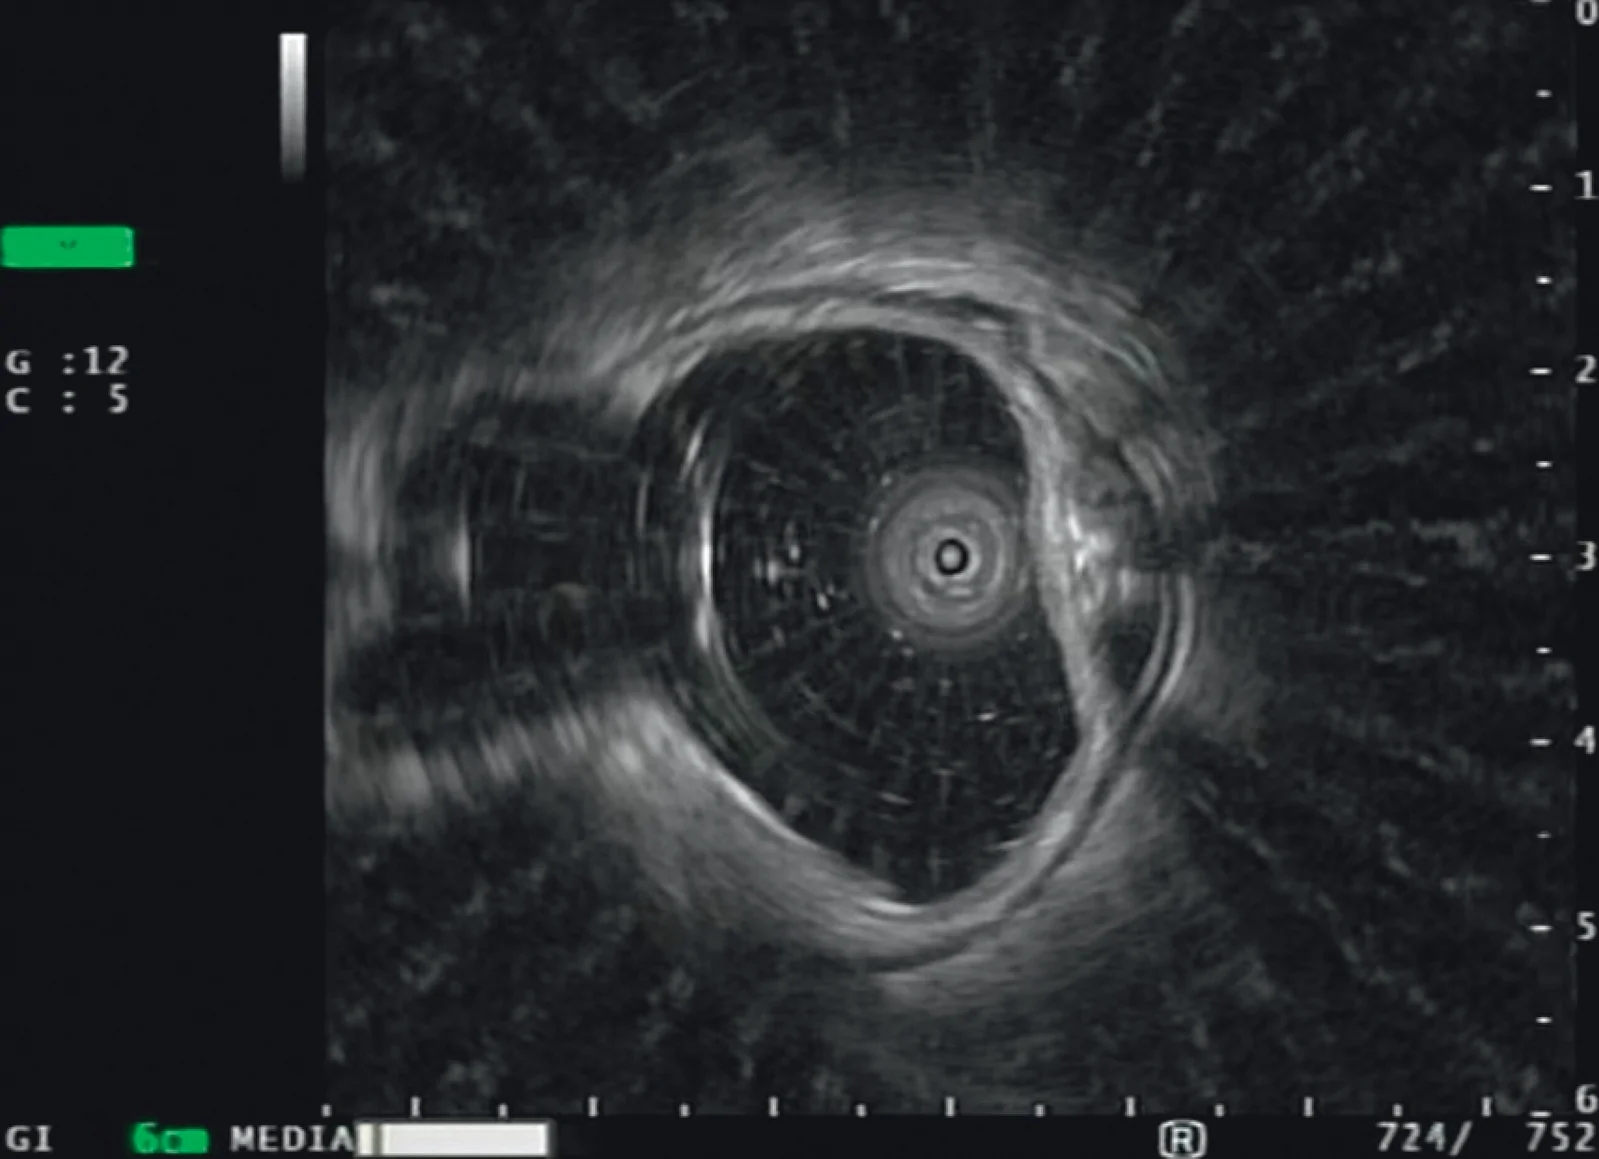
Endoscopic ultrasonography (EUS) revealed a hyperechoic focus
